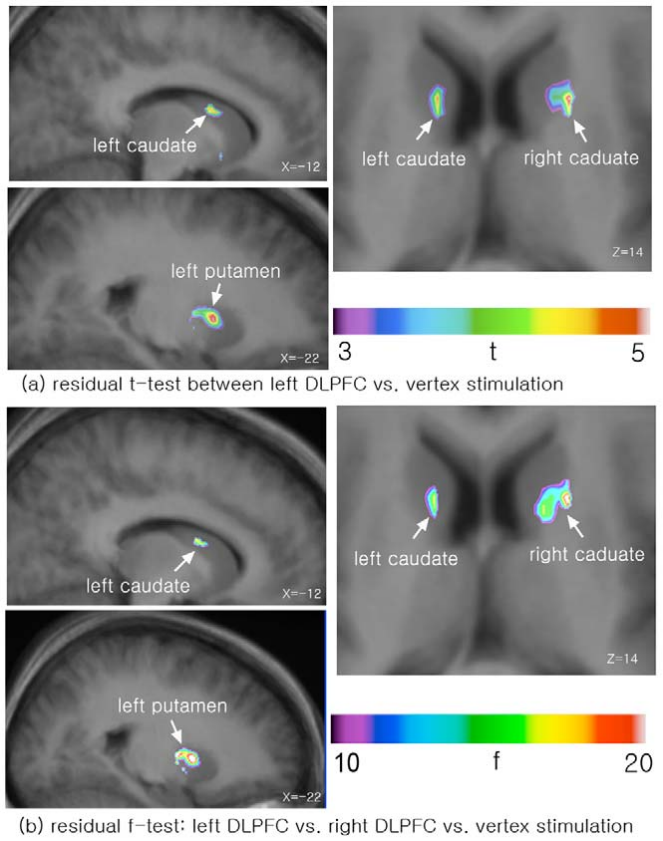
The similar results of t-test and f-test on real data. (a) t-test between left DLPFC stimulation vs. vertex stimulation (control condition): The t-map is overlaid upon the averaged MRI of all subjects in standard MNI space (published with permission from Ko et al. [9]). (b) f-test of all three stimulation condition (left DLPFC, right DLPFC and vertex stimulation): As the right DLPFC stimulation had no significant effect on BP in these regions, the f-map shows the similar pattern of t-map of left DLPFC vs. vertex stimulation.
